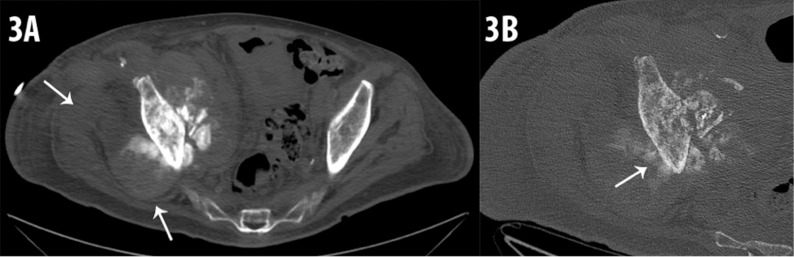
(A) Axial CT image of the pelvis. There is a soft tissue mass centered in the right acetabulum with dense sclerosis within the iliac bone, osteoid matrix and aggressive extension into the adjacent iliacus and gluteal musculature (white arrows). (B) High resolution axial CT with magnification of the right acetabulum. The destructive nature of the tumor within the cortex of the iliac bone is clearly defined. Osteoid matrix within the soft tissue component (white arrow). CT- Computed Tomography
